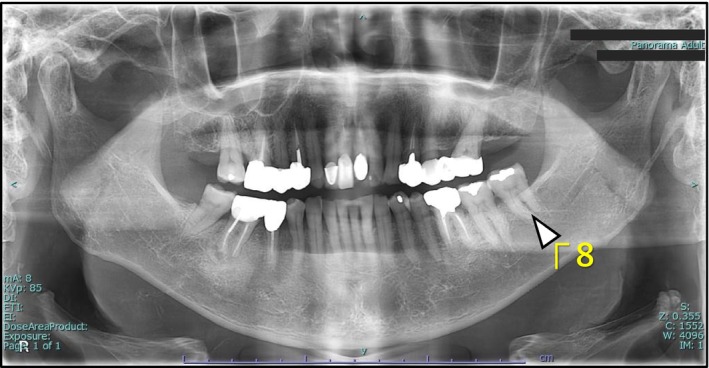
The preoperative orthopantomogram indicates the erupted third molar in the left mandible which was the cause of pericoronitis (white arrow).
